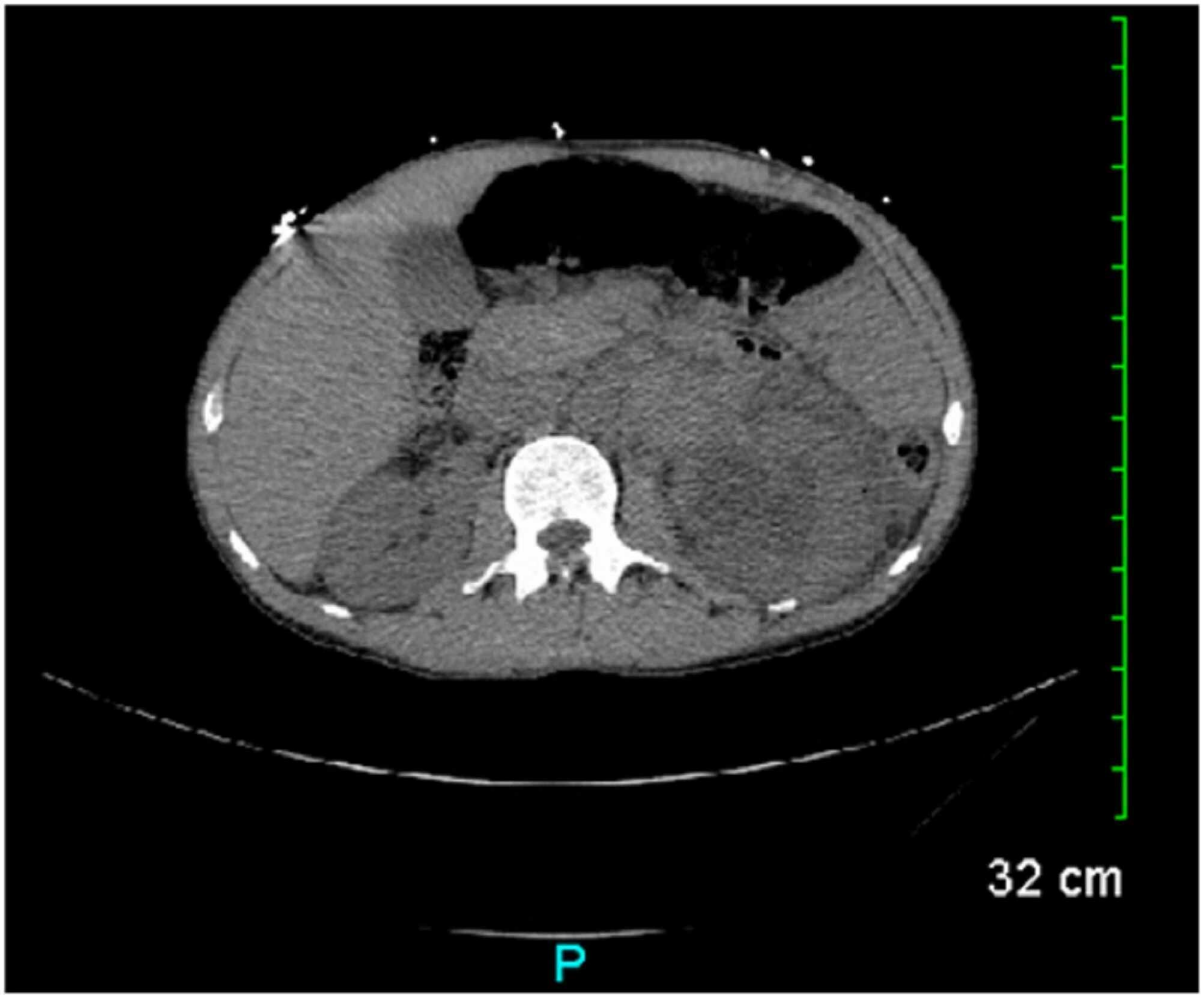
CT scan of the abdomen revealing central necrotic mass measuring 7 x 5.4 x 9 cm with mass-effect
